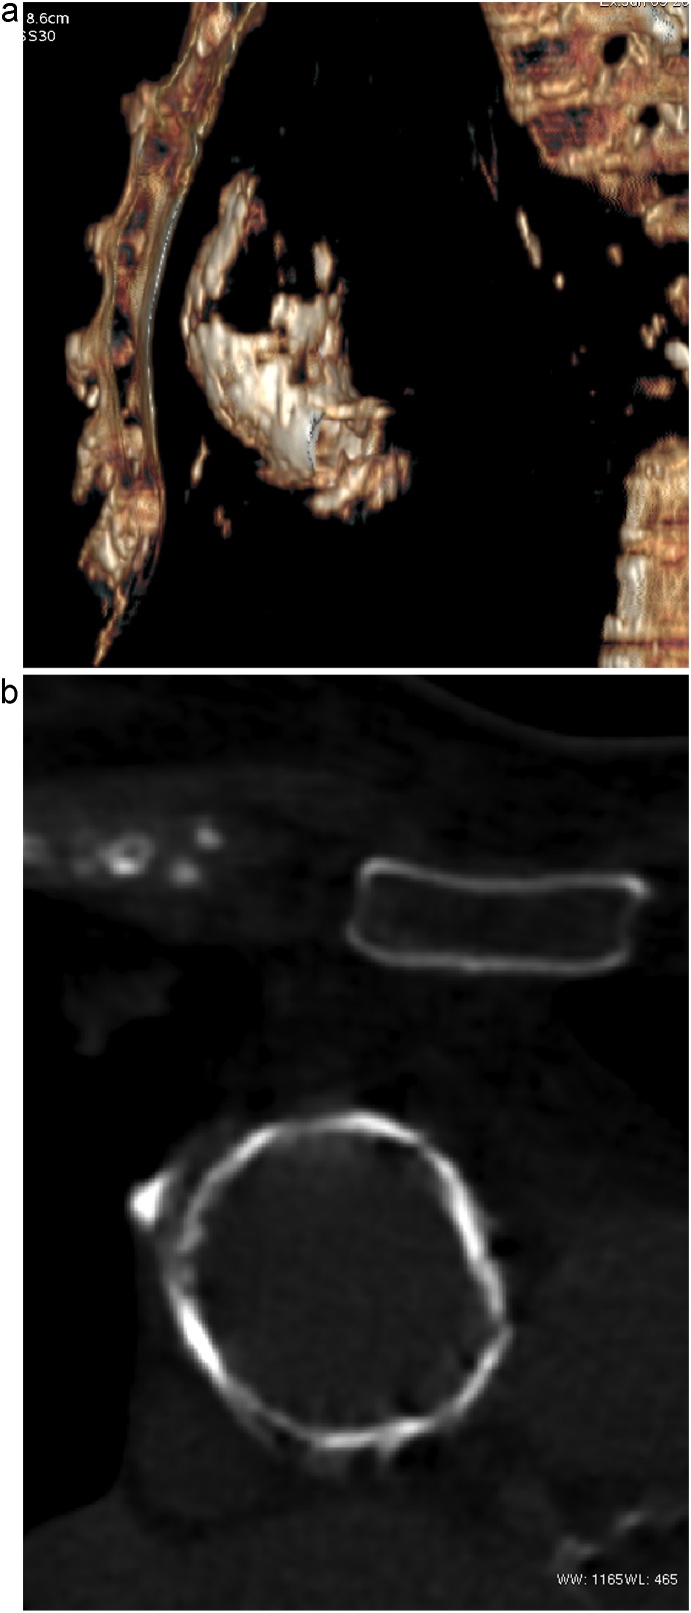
Computed tomography of the thorax that confirms a porcelain aorta: 2a scan volume rendering reconstruction, 2b: cross sectional view of contrast enhanced CT.
